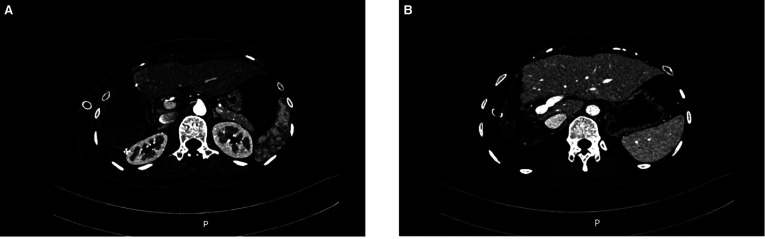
Postoperative CT findings. (A) Arterial phase (B) Venous phase.
